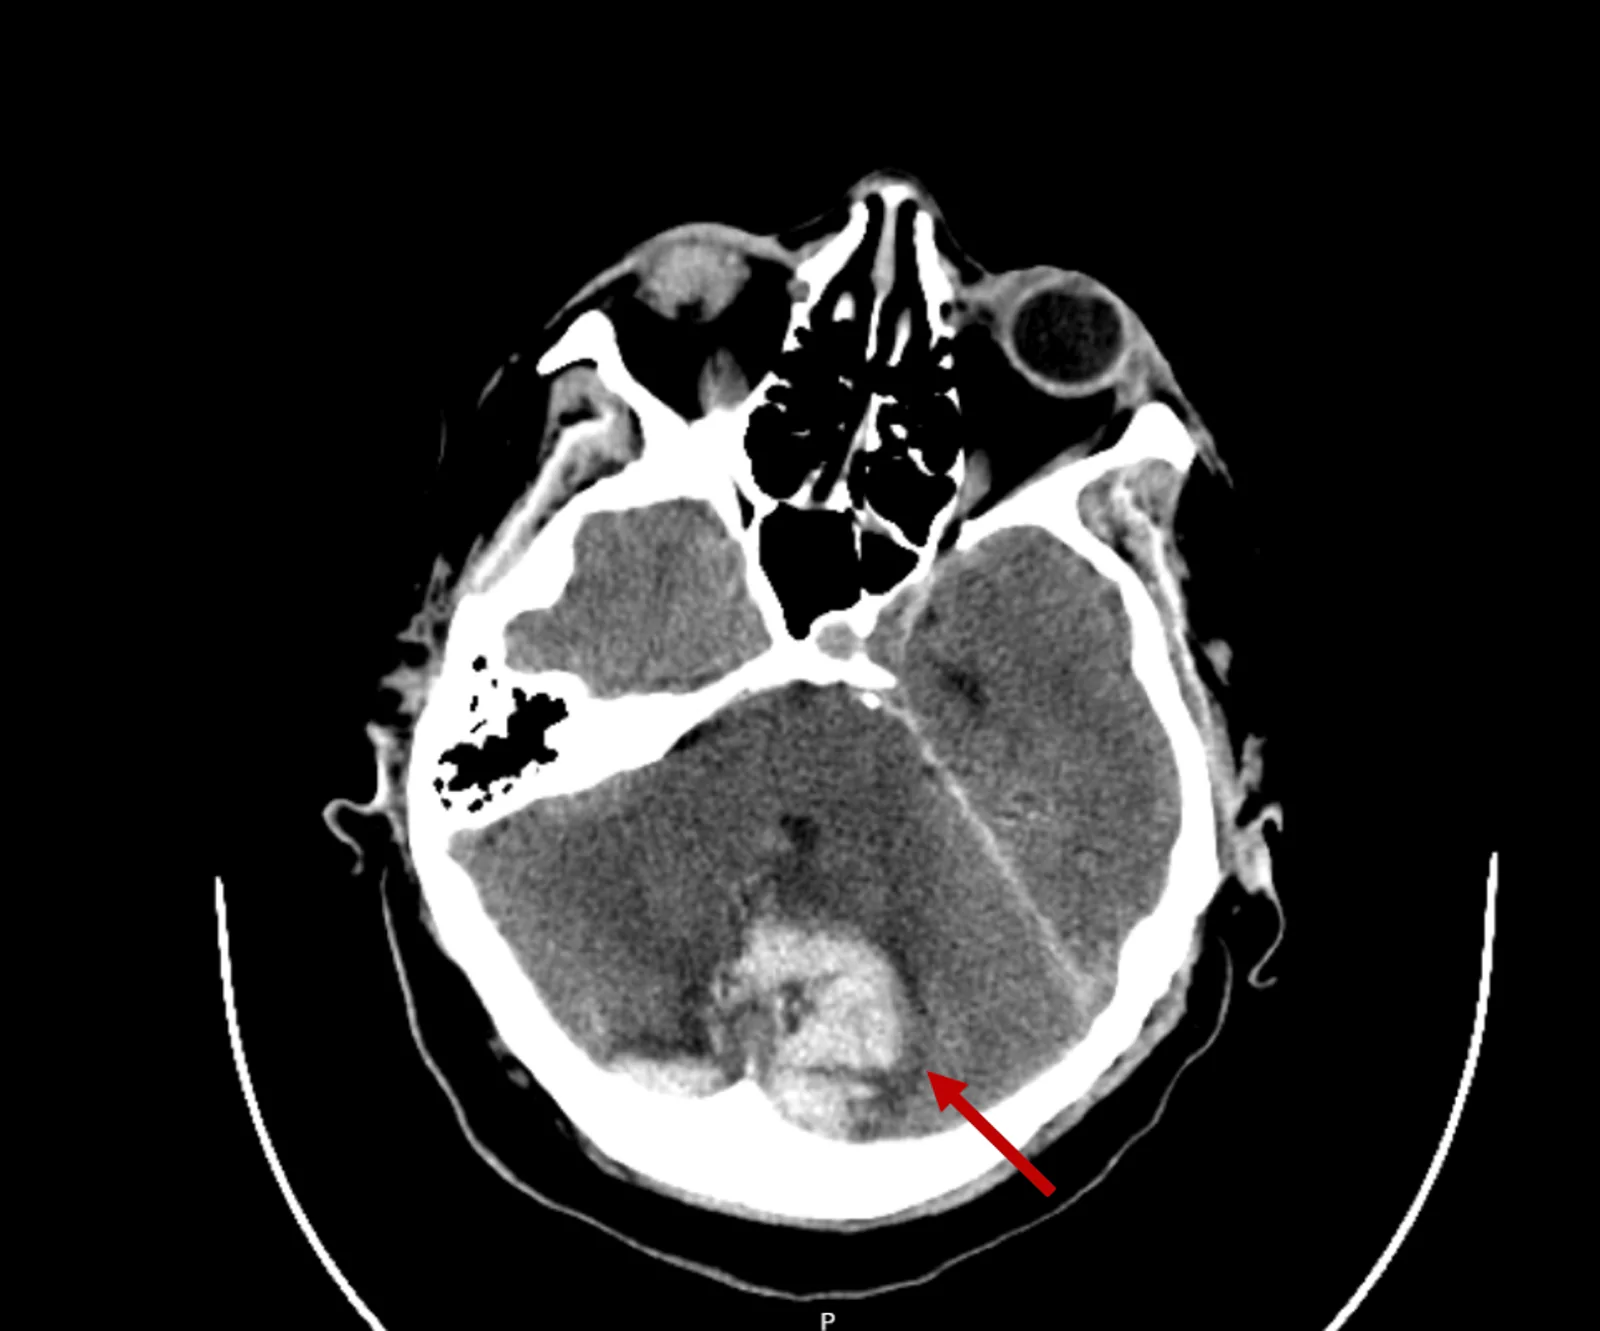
Axial CT scan of the brain Acute left cerebellar haemorrhage (arrow) with mass effect.
